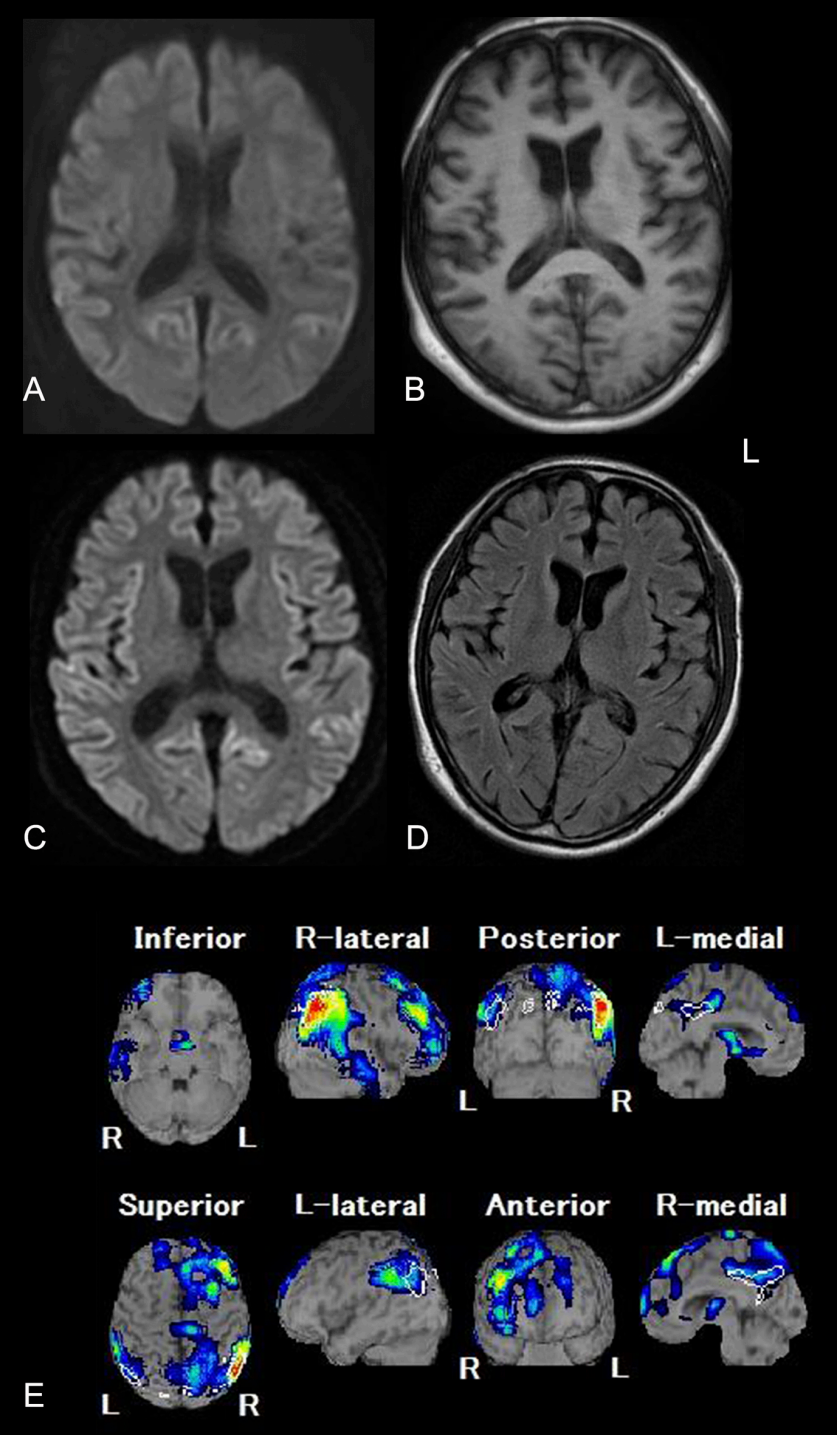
Neuroimaging of the present case. A. Diffusion weighted imaging (DWI) obtained on April 2013. Mild hyperintense lesions in the parietal and posterior lobes. B. T1-weighted image obtained on June 2013. Mild cerebral atrophy is present. DWI was not performed at the same time. C, D. Magnetic resonance imaging (MRI) obtained on December 2013. DWI (C) shows hyperintense lesions in cortical ribbons and caudate nuclei. No apparent signal abnormalities are present in fluid-attenuated inversion recovery (FLAIR) images (D). E. ^99m^Tc-ECD SPECT combined with the easy Z-score imaging system (eZIS) (July 2013) shows hypoperfusion at the level of the frontal, temporal, and parietal lobes, including the precuneus and posterior cingulate gyrus.
